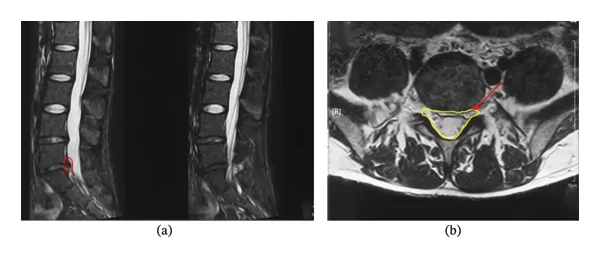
Long‐term postoperative follow‐up MRI at 6 months. (a) Sagittal fat‐suppressed T2‐weighted sequence; the red circle highlights the completely restored patency and vertical continuity of the central spinal canal at the L5–S1 index level. (b) Axial fat‐suppressed T2‐weighted sequence; the total cross‐sectional area of the spinal canal is traced/outlined, and the red arrow indicates the thoroughly decompressed nerve root with an anatomically normalized dural sac profile and clear perineural padding.
